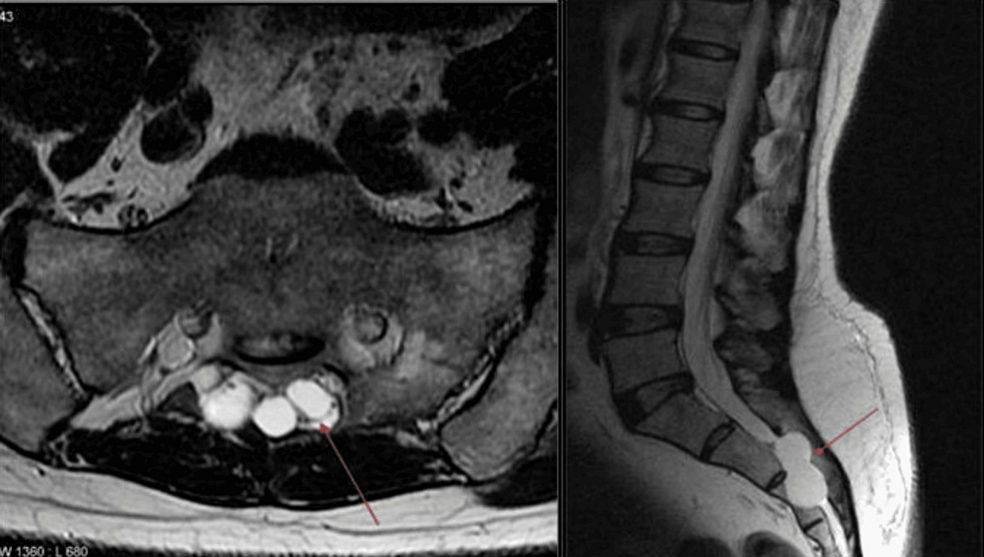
Axial and sagittal MRI T2 images of the spine showing perineural cysts. Image source: Case courtesy of Frank Gaillard, Radiopaedia.org. From the case https://radiopaedia.org/cases/6284?lang=gb rID: 6284
